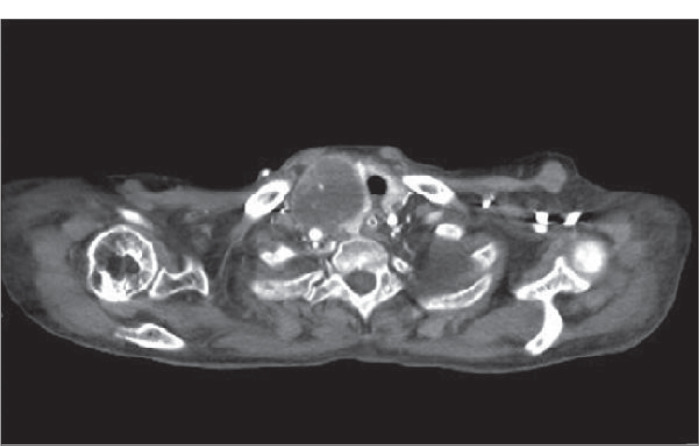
Computed tomography of thyroid mass.
